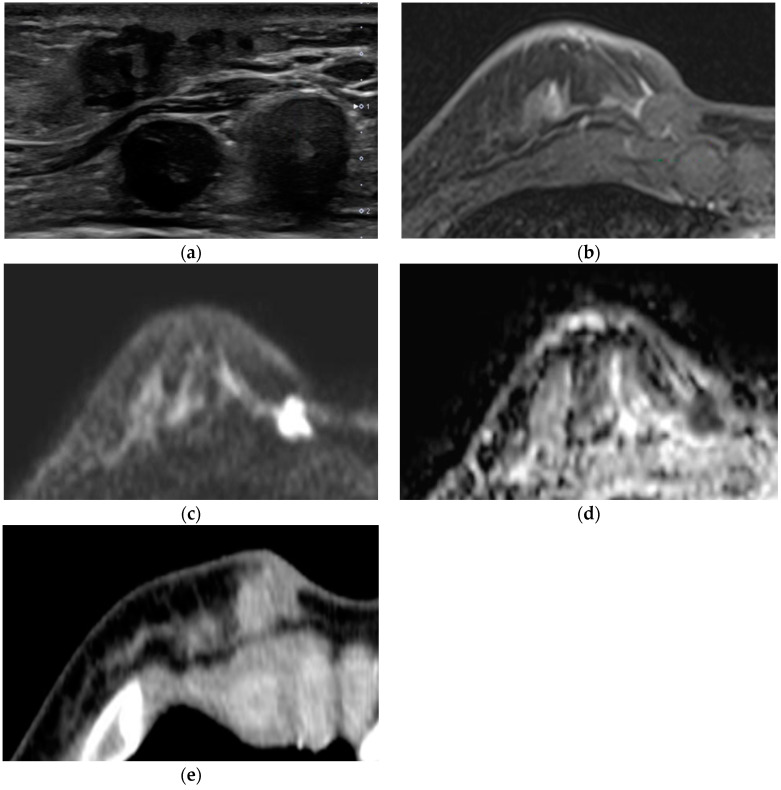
A 42-year-old woman with palpable mass at breast periphery. (a) In the ultrasound, a 2.3 cm irregular hypoechoic mass was detected in the palpable site of the right breast at the 4-o’ clock periphery and confirmed as invasive ductal carcinoma by core needle biopsy. (b) No discernible enhancing lesion at the correlating site in the early phase of dynamic contrast-enhanced T1WI. This palpable mass showed definite diffusion restriction with an ADC value of 0.68 × 10^−3^ in DWI b = 1000 (c), ADC map (d), and homogeneous enhancement in contrast-enhanced chest CT in the supine position (e).
